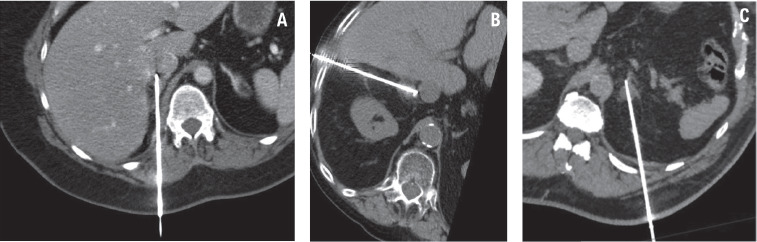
Intraprocedural reconstructed native or contrast-enhanced computed tomography (CT) images showing the microwave antennas within the adenomas: in the right adrenal gland of the case 1 patient (A), in the right adrenal gland of the case 2 patient (B) and in the left adrenal gland of the case 3 patient (C).
